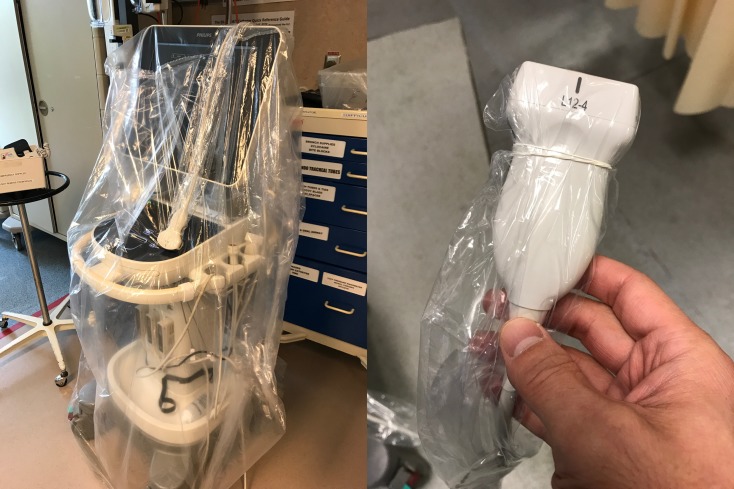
With AGMP, cover non-essential components of the ultrasound machine with drapes, gown, or plastic covering, and use sheaths to cover the probe and cord.
